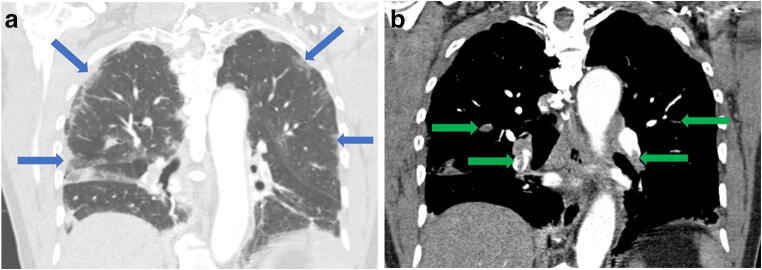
Early stage COVID-19 (0 to 4 days) with acute pulmonary embolism: a Coronal CT scan of the chest (lung window) in a 79-year-old man with COVID-19 demonstrates peripheral ground glass opacities in subpleural distribution (blue arrows). b Coronal CT scan of the chest with IV contrast (soft tissue window) demonstrates filling defects within the right and left pulmonary arteries (green arrows), indicating acute pulmonary embolism.
